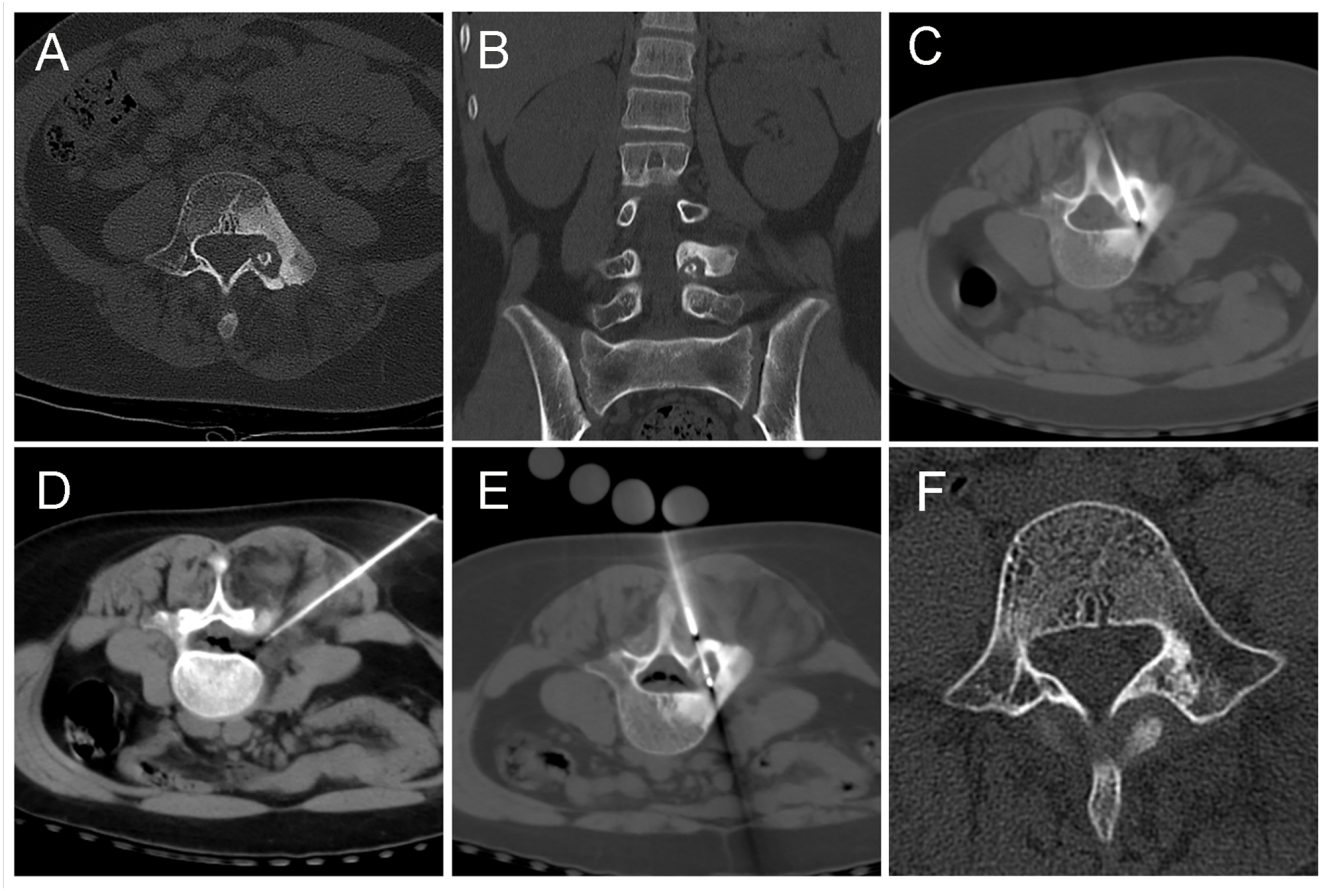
An 11-year-old girl with low back pain, predominantly at night. (A, B) The lesion was mainly located in the left pedicle with marked hyperplastic sclerosis of the bone around the lesion. (C, D, E) The patient was positioned prone, and the freezing probe was inserted from the back, directly through the center of the lesion. The fine needle was used to inject air into the epidural space to protect the intravertebral structures. (F) One year after the procedure, a follow-up CT scan showed that the former low-density lesion area had been replaced by high-density bone tissue, and the osteosclerotic area around the lesion had surprisingly returned to normal on this review.
